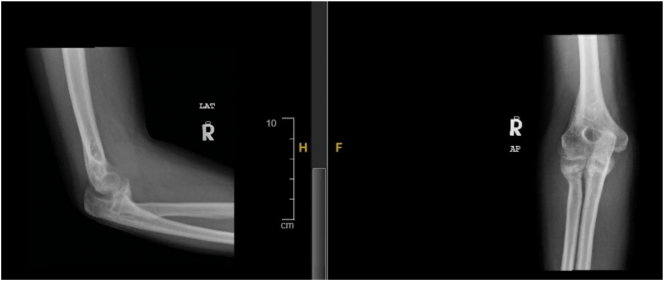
Represents the elbow of the patient showing dislocated joints. X-ray showing dislocation of the right elbow at the first presentation.
